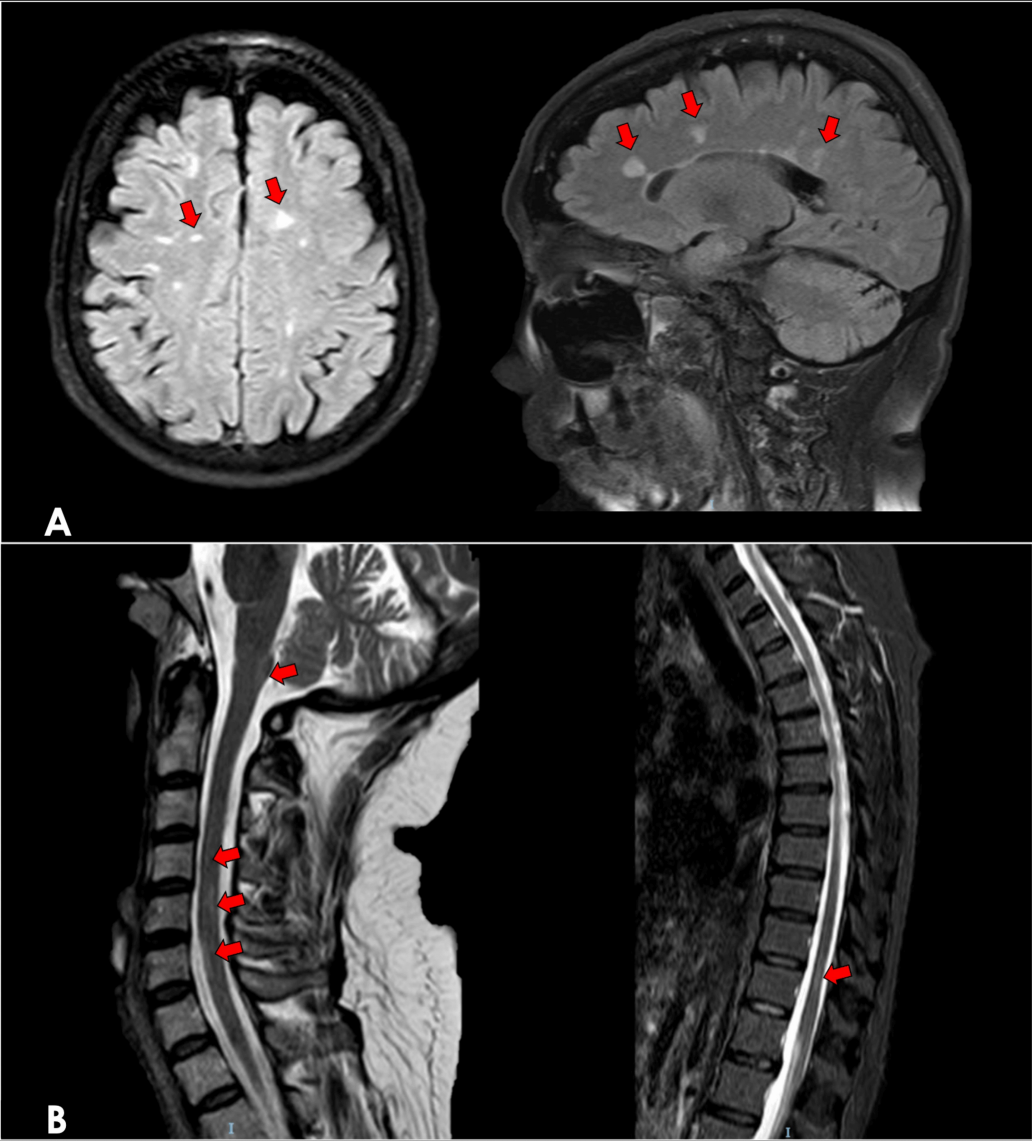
MRI done two months prior to aHSCT (February 2023) (A) Representative axial and sagittal brain MRI images show multiple FLAIR hyperintense lesions over the juxtacortical, subcortical, and periventricular areas with mild global cerebral atrophy. (B) Representative sagittal spinal MRI images show T2 hyperintense lesions over the posterior cervicomedullary junction, cervical spinal cord (C4-C5 and C6-C7), and thoracic spinal cord (T10-T11) with mild cord atrophy. In comparison to previous brain and spinal MRI procedures, these findings are stable and show no radiologic progression. aHSCT - autologous hematopoietic stem cell transplantation; FLAIR - fluid-attenuated inversion recovery
